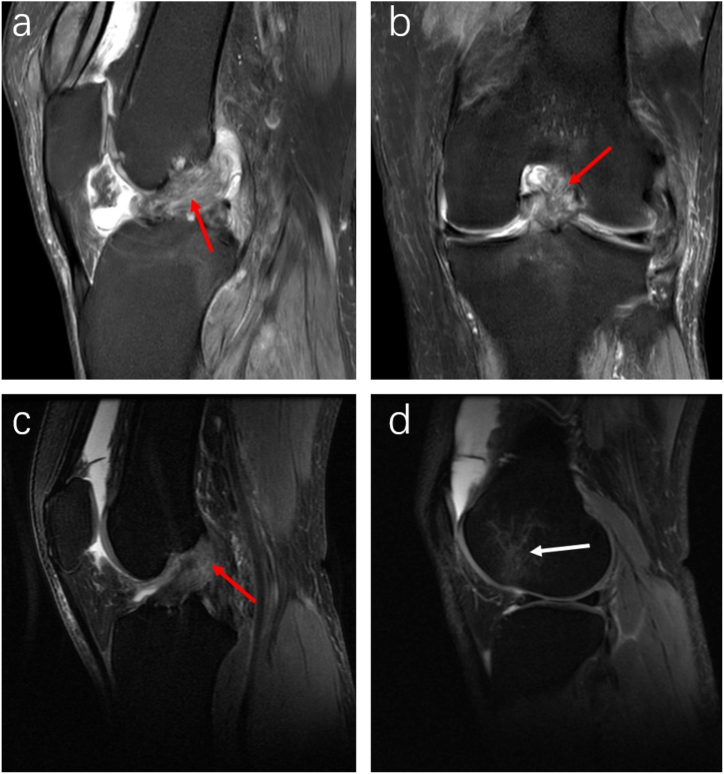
MRI features of severe ligament involvement (a, b). A 50-year-old male patient with a 23-year history of KGA exhibits complete disarray of ACL fibers on the sagittal and coronal PDWI-FS sequence. The sequence shows uneven signals and diffuse intermediate-to-high mixed signals marked by a red arrow, and “fibrous root-like” changes. MRI images indicate a combination of KGA and an ACL tear (c, d). Similarly, a 32-year-old male patient with KGA and an ACL tear displays a significantly curved and thickened ACL with increased internal signal intensity on the sagittal PDWI-FS sequence. The sequence reveals multiple patchy, slightly long T2 signal changes, and an irregular ACL femoral insertion point indicated by a red arrow. The fiber course direction and angle are diminished. Kissing contusions on the lateral femoral condyle are marked by a white arrow, presenting as a slightly high T2 signal with a diffuse distribution.
